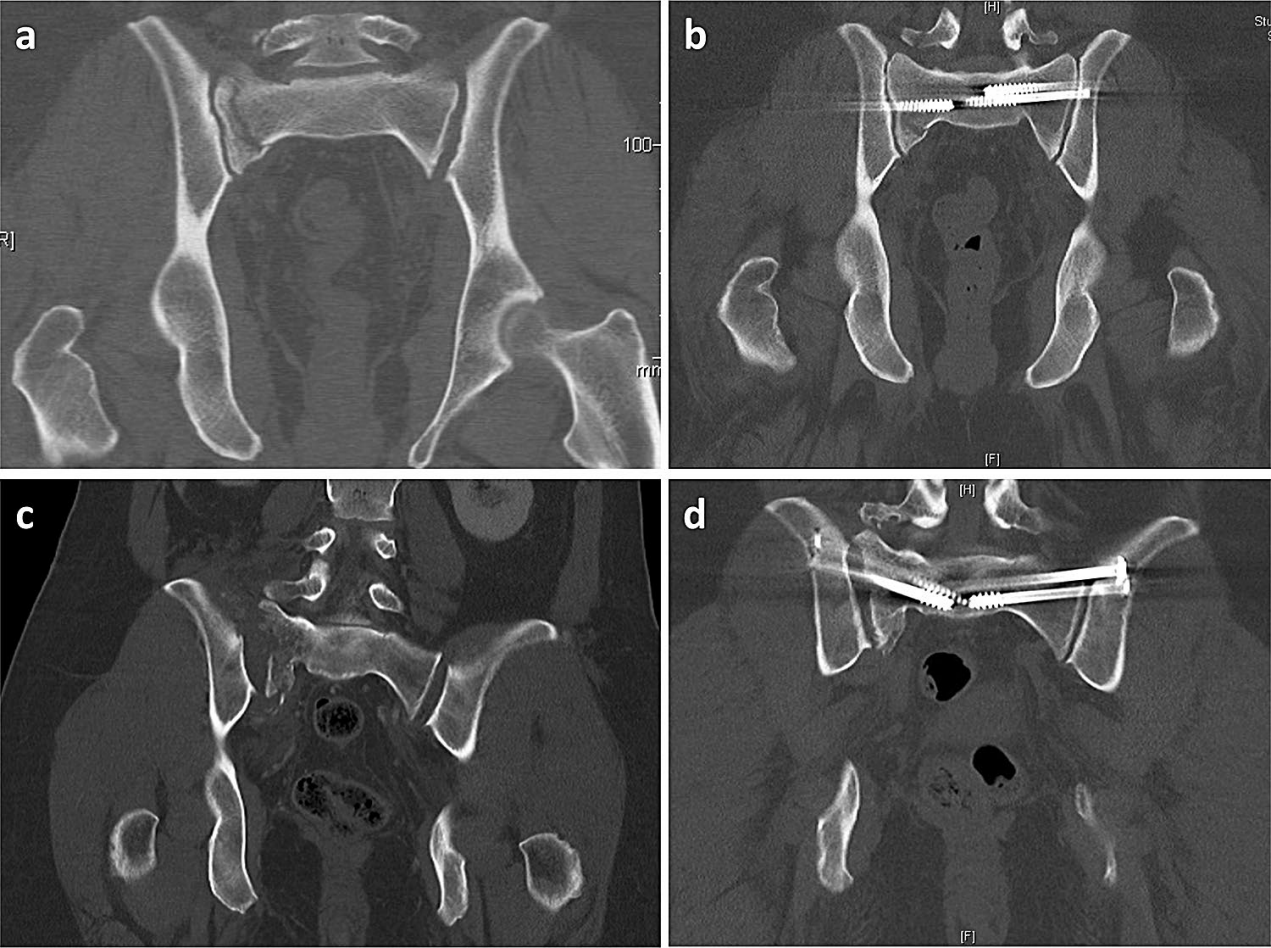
CT picture of two patients showing bilateral pelvic injuries before and after the surgery. a CT picture (coronar view) of an injured pelvis of a patient before and b after the surgery with anatomic reduction. c CT picture (coronar view) of an injured pelvis of a patient before and d after the surgery without anatomic reduction
